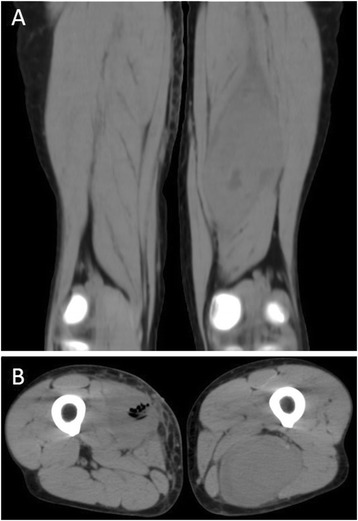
CT scan views of the thigh abscesses. The infection presents as an isodense image located within the muscles with a small necrotic centre. a: coronal view of the left thigh abscess. b: axial view of the left and right abscesses. The presence of air in the right thigh abscess is due to a wick that was left to ensure drainage of the abscess
